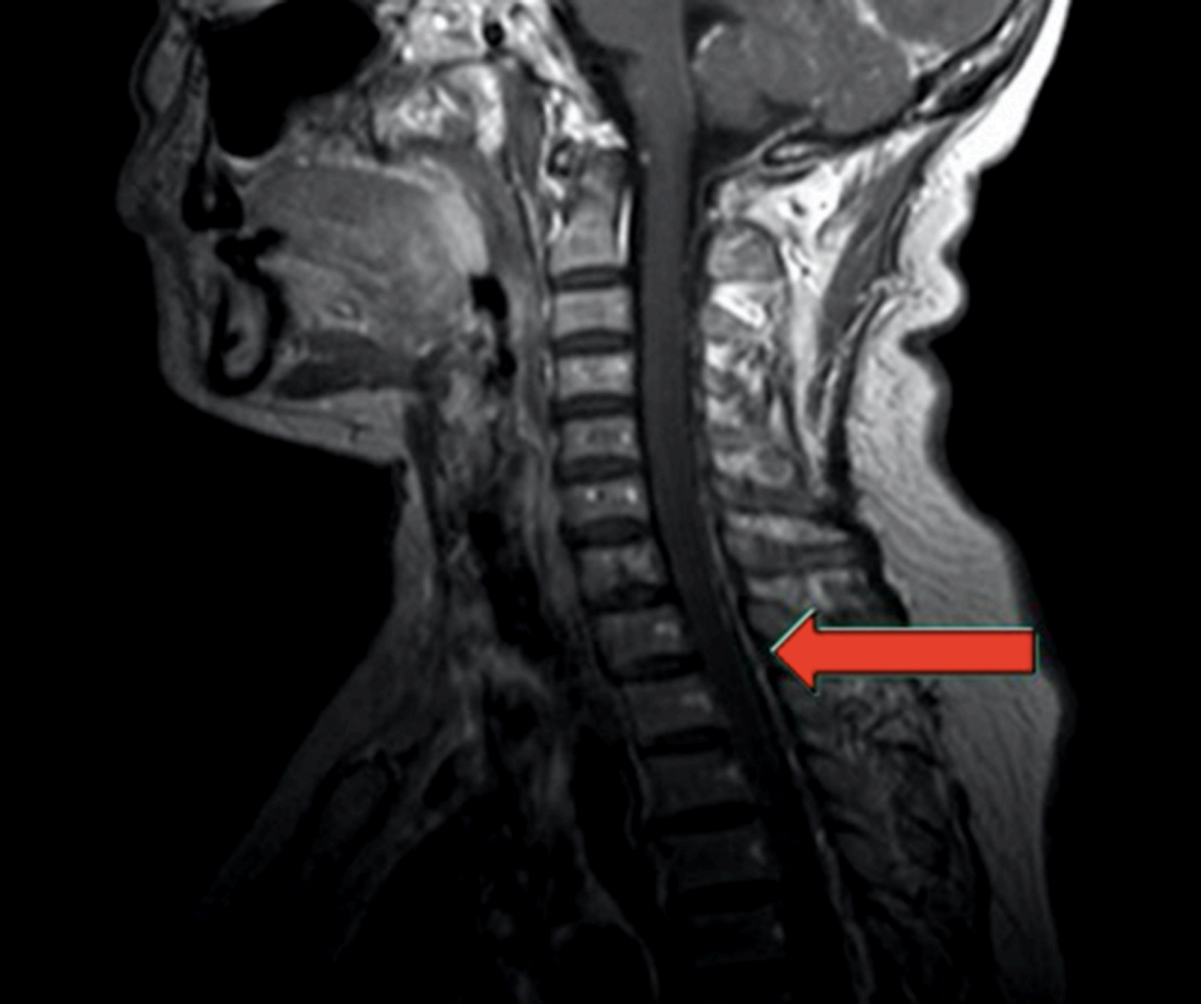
Magnetic resonance image of the same patient The image showed metastasis of spinal dura mater, which meant a failure in maintenance treatment and demand for shift of therapeutic regimen.
